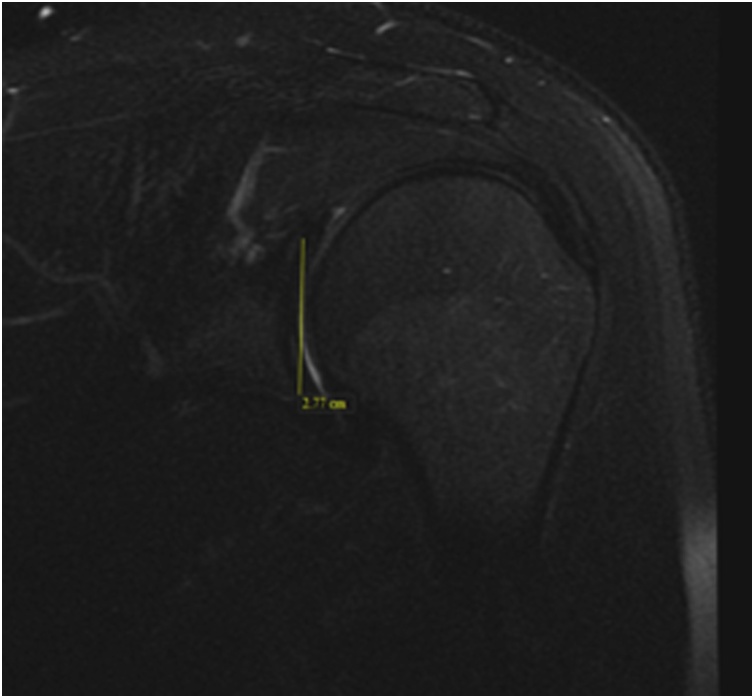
Glenoid height in the coronal view on shoulder MRI.
